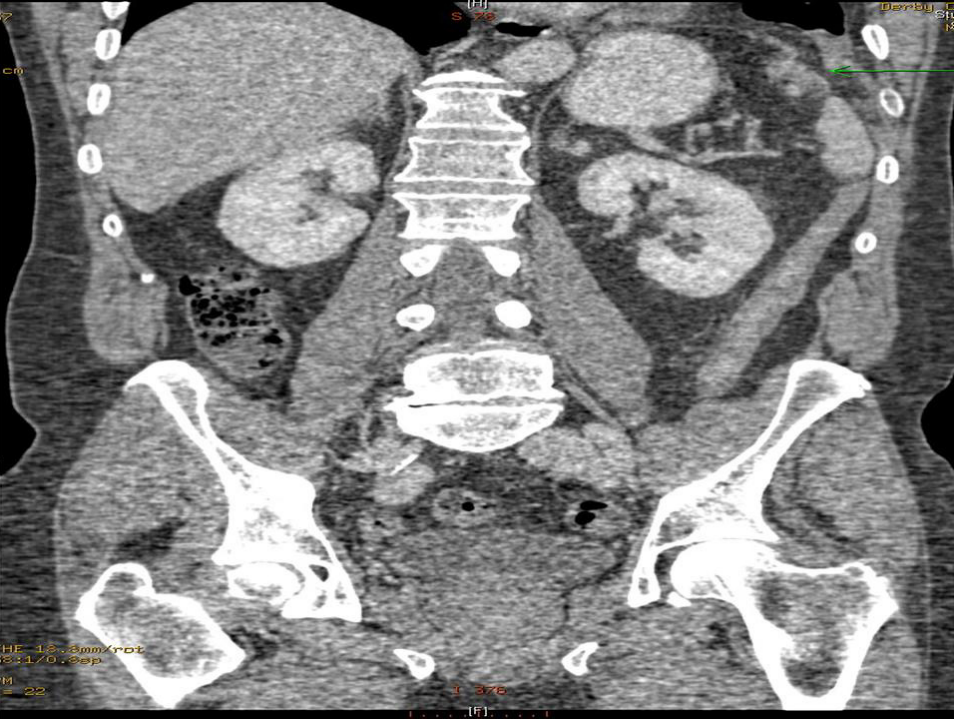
Coronal CT scan showing disrupted left hemidiaphragm.
